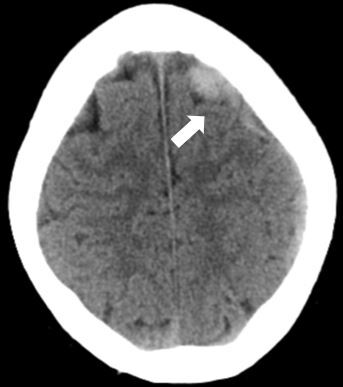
Transverse section of a computed tomography image of the head showing an acute subdural hematoma along the left frontal cerebral hemispheric convexity (arrow).
